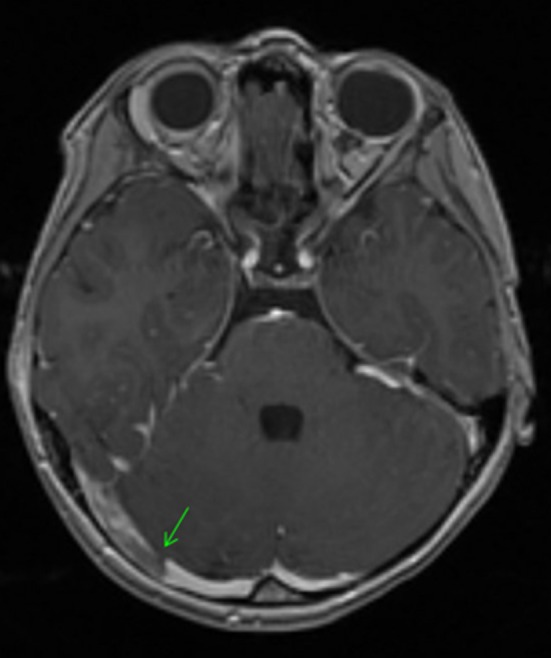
MRI with contrast performed 10 d following initial imaging. Arrow indicates the thrombus within the transverse sinus. Interval decrease in size as indicated by the contrast now visualized beyond the thrombosis
